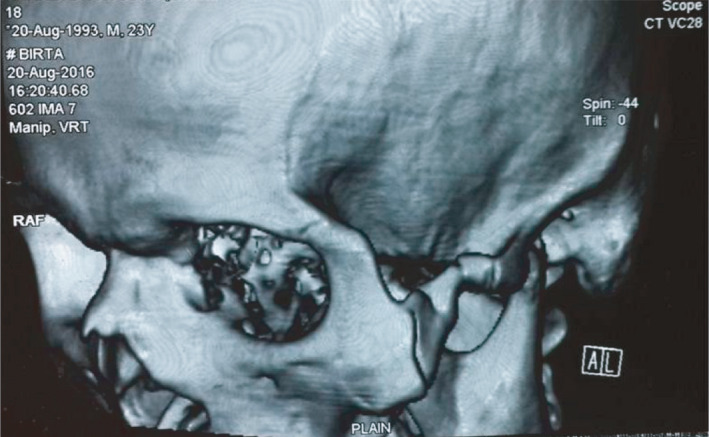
Pre‐operative 3D view of CT scan of same patient
